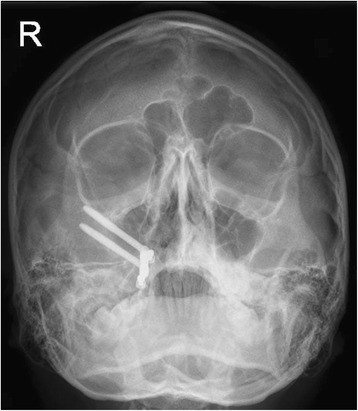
Facial radiograph at 22-month follow-up
